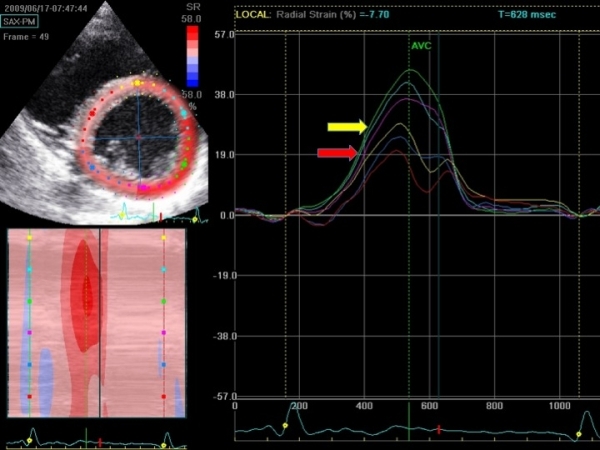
Radial strain assessment using 2D speckle tracking echocardiography. Radial two-dimensional strain in the parasternal short-axis view at the mid-ventricular level of a child with a septal accessory pathway and LV dysfunction. There is an inhomogeneous pattern of radial strain, with severely reduced peak radial strain values for septal myocardial segments (yellow and red arrows). Radial peak strain values are presented as positive values.
